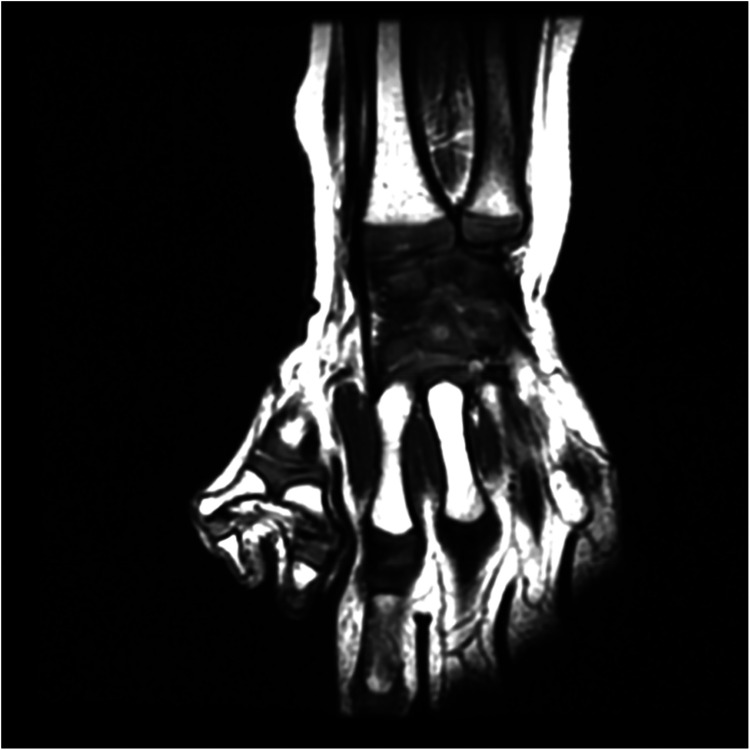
Preoperative MRI showing the angular deformity. The MRI provides detailed visualization of the cartilaginous structures and soft tissues.
